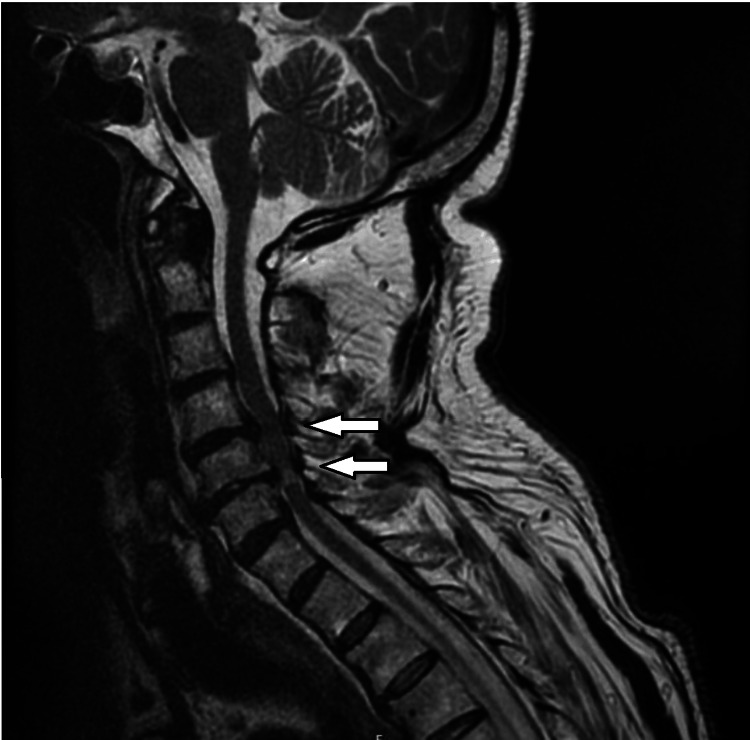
Cervical MRI Arrows: medullary narrowing at C4-C5 and cervical spondylotic myelopathy centered behind the C5-C6 disc
